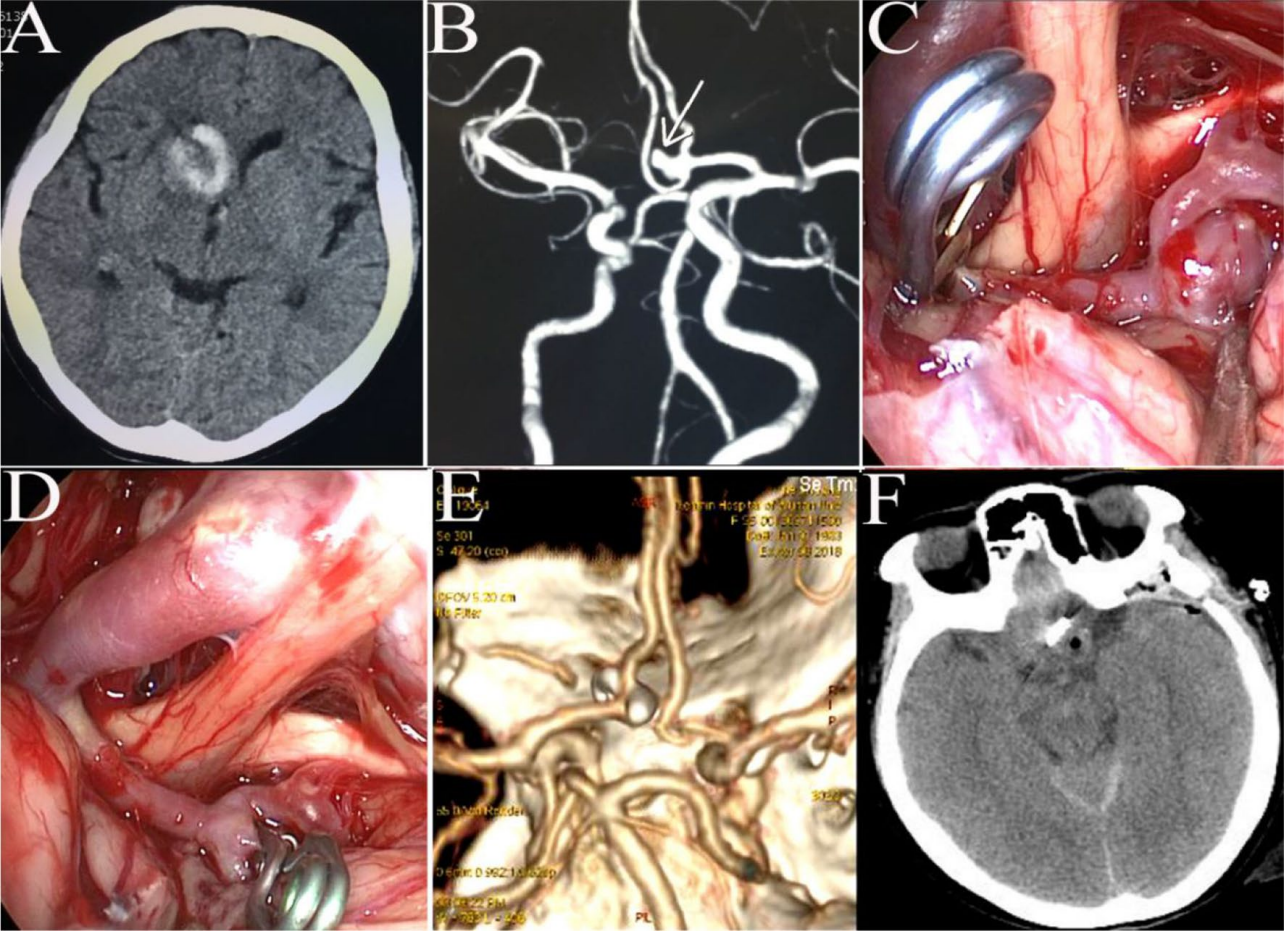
An ACoA aneurysm was clipped under neuroendoscopy. (A) Preoperative CT showed a small hematoma in the basal ganglia and right lateral ventricle. (B) Preoperative CT angiography (CTA) showed a saccular aneurysm at the ACoA complex. (C) Left A1 was clipped temporarily, and the aneurysm, as well as the left and right A2, were exposed. (D) The aneurysm was clipped under neuroendoscopy, and the temporary clip was removed. (E) Postoperative CTA showed complete clipping of the aneurysm. (F) Postoperative CT showed proper placement of the aneurysm clip.
